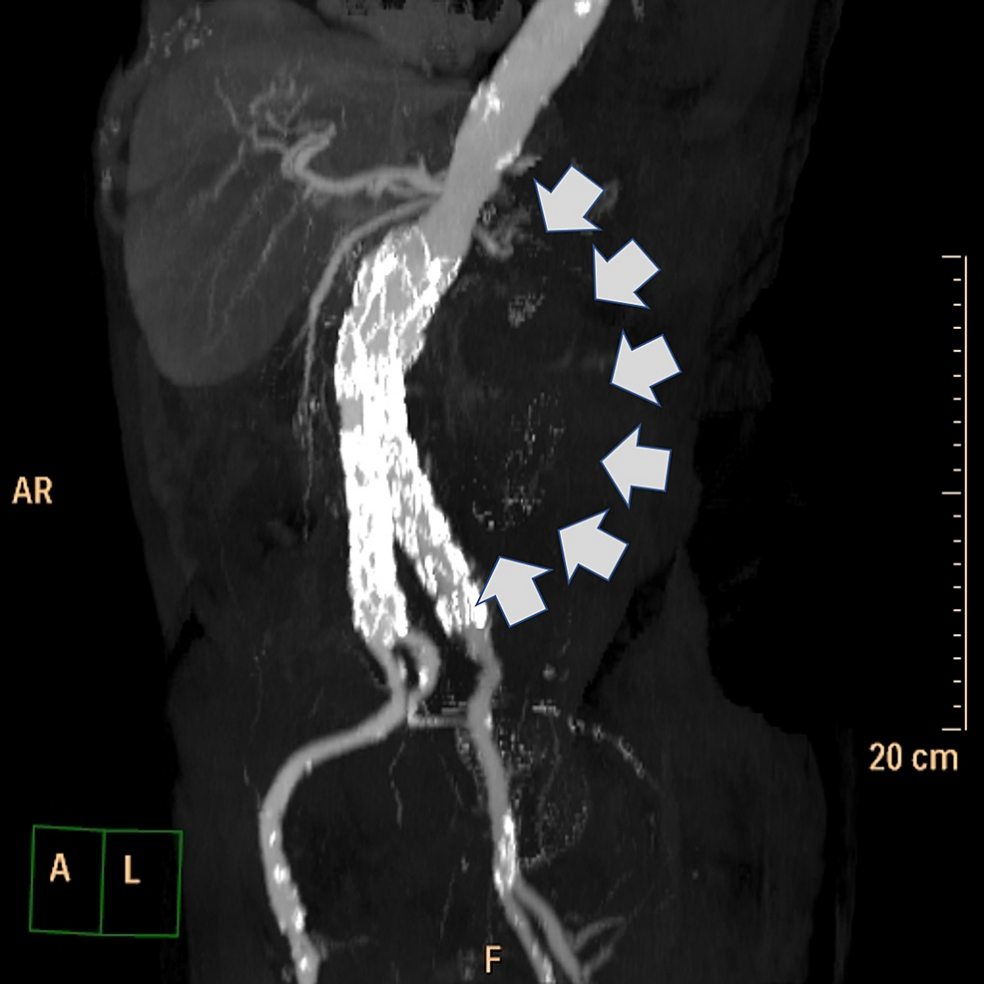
Pre-operative CT angiography of the patient. Computed tomography angiography (3D reconstruction) depicting the escape of the contrast media from the left iliac branch of the endovascular stent to the retroperitoneal mass under investigation (grey arrows).
